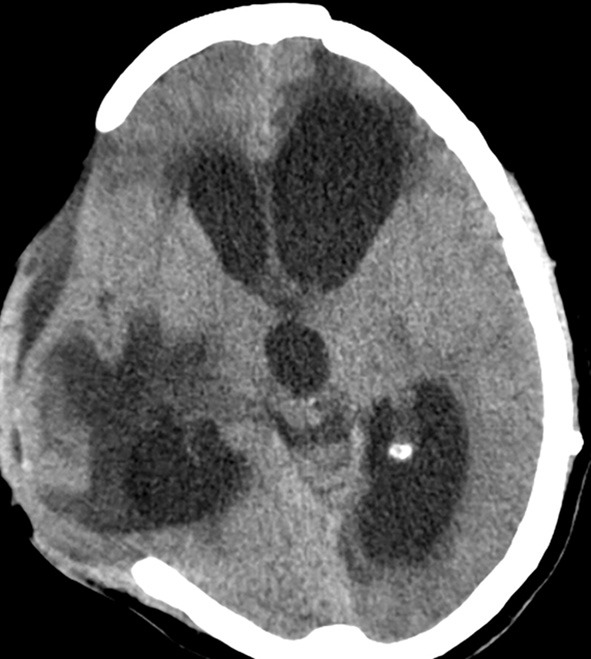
Hydrocephalus. Computed tomography depicting a case of hydrocephalus after craniectomy.
